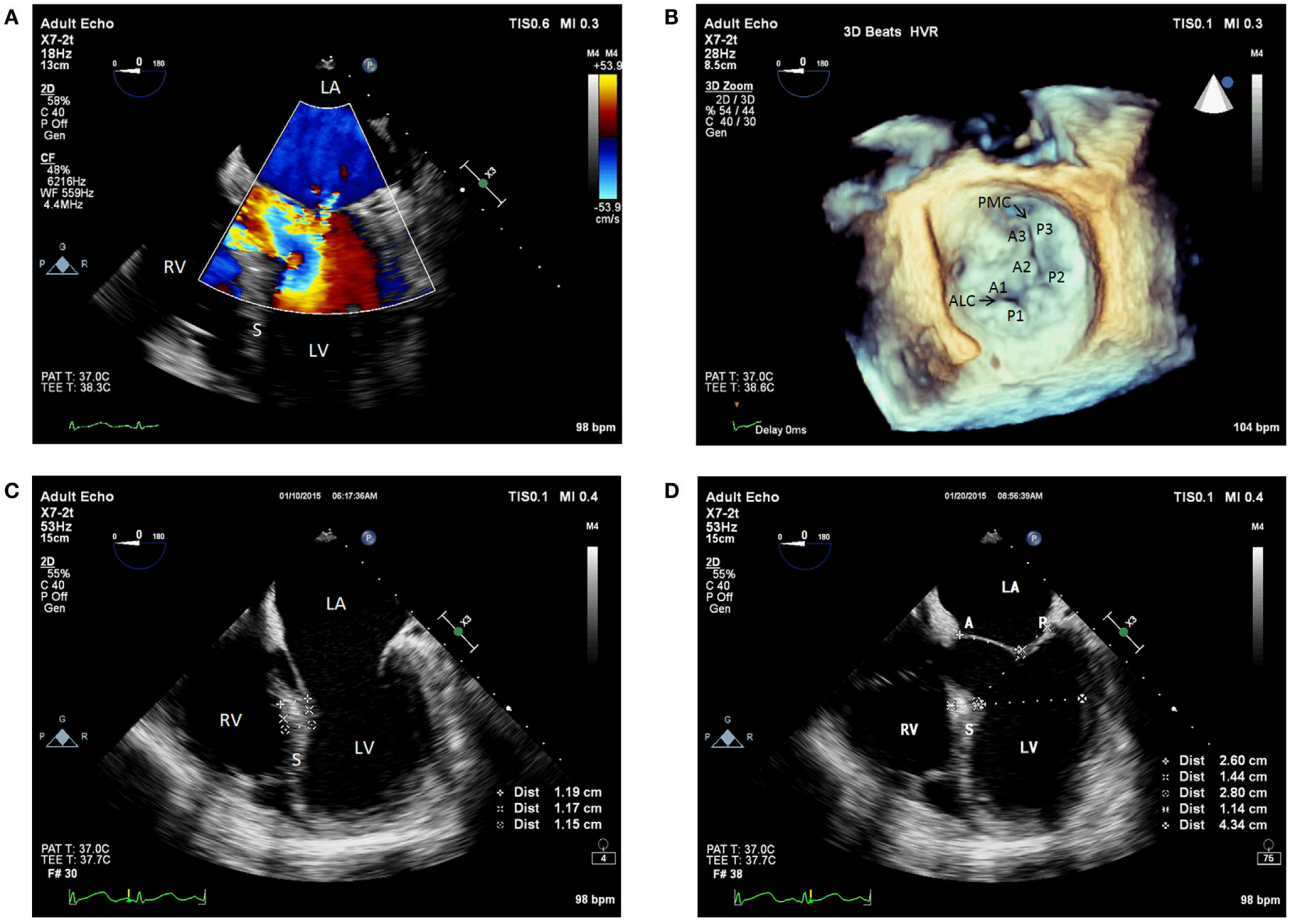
(A) Pre-anhepatic 2D TEE midesophageal modified five-chamber view with color flow Doppler showing trace to mild mitral regurgitation. (B) Pre-anhepatic 3D TEE en face view of the mitral valve visualized from the left atrial perspective indicating normal coaptation of the mitral leaflets during systole. (C) Pre-anhepatic 2D TEE modified five-chamber view in diastole demonstrating mild basal interventricular septal hypertrophy. (D) Pre-anhepatic 2D TEE modified five-chamber view in systole demonstrating the absence of risk factors for systolic anterior motion of the mitral valve leaflets. Absent risk factors include normal values for basal LV systolic dimension (4.3 cm), ratio of anterior to posterior mitral leaflet coapted length (1.86), and distance between the mitral coaptation point and the basal LV septum (2.8 cm). LV, left ventricle; RV, right ventricle; LA, left atrium; S, interventricular septum; ALC, anterolateral commissure; PMC, posteromedial commissure; A, anterior mitral valve leaflet; A1, first segment of the anterior mitral valve leaflet; A2, second segment of the anterior mitral valve leaflet; A3, third segment of the anterior mitral valve leaflet; P, posterior mitral valve leaflet; P1, first segment of the posterior mitral valve leaflet; P2, second segment of the posterior mitral valve leaflet; P3, third segment of the posterior mitral valve leaflet.
